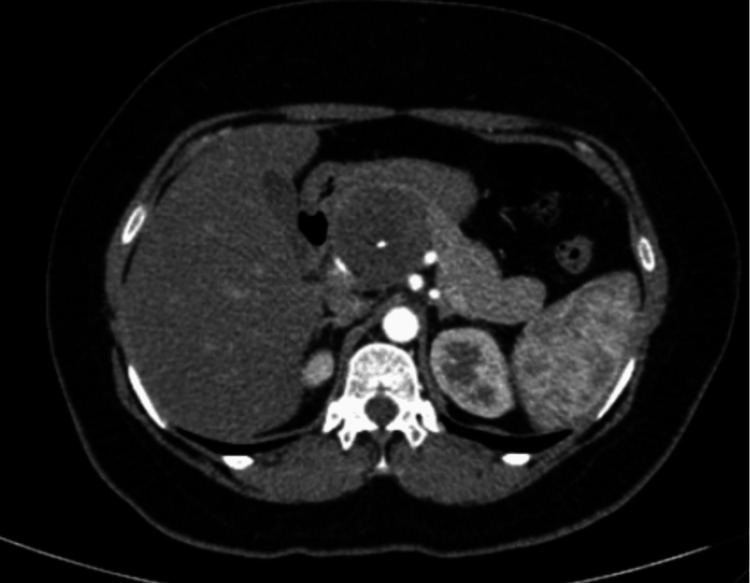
Arterial phase of CT scan showing the retroperitoneal tumor arising from the head of pancreas and blood vessels
